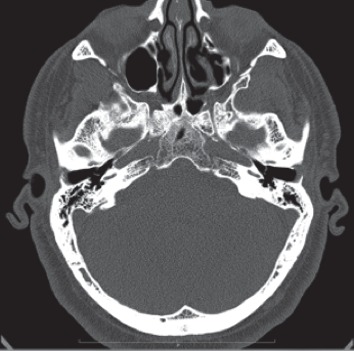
The computed tomography (CT) did not show bone alterations or changes suggestive of malignant external otitis.
